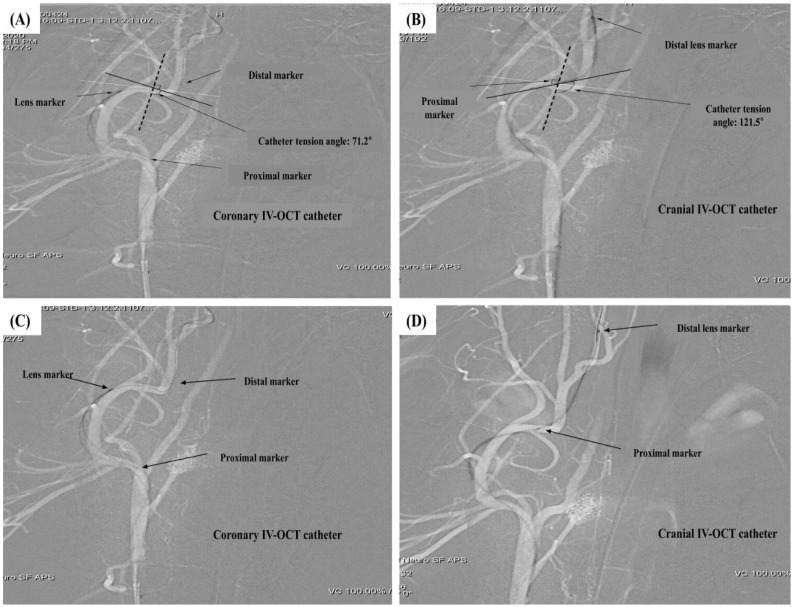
Preclinical evaluation of modified cranial IV-OCT catheter trackability and pushability in the external carotid artery (ECA). (A,B) Catheter tension angle (CTA) was used to assess trackability. CTA was defined as the angle between the vertical line (black dashed line) from the center of the parallel line to the horizontal parent artery (white line) and the distal catheter line (black line). Measured CTA values were 71.2° for the coronary IV-OCT catheter and 121.5° for the cranial IV-OCT catheter. (C,D) Pass of catheter (POC) was used to assess pushability. POC was defined as the distal advancement of the catheter beyond the 4th ECA curve. Non-pass was defined as no further distal movement. Coronary and cranial IV-OCT catheters showed non-passing and successful passing, respectively, of the distal catheter tip beyond the 4th curve.
